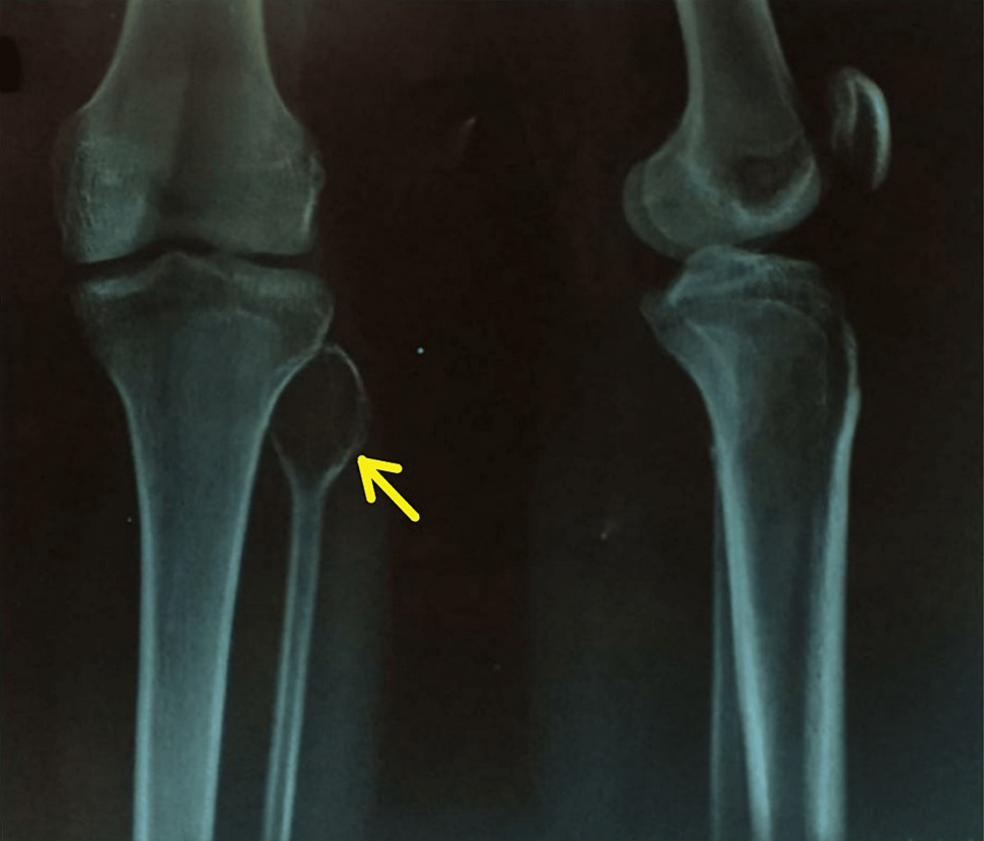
X-ray of the right knee with proximal one-third of the right tibia and fibula showing the radiolytic shadow and pencil-thin cortices of the head of the right fibula. The arrow marks the expansile ballooning lesion.
